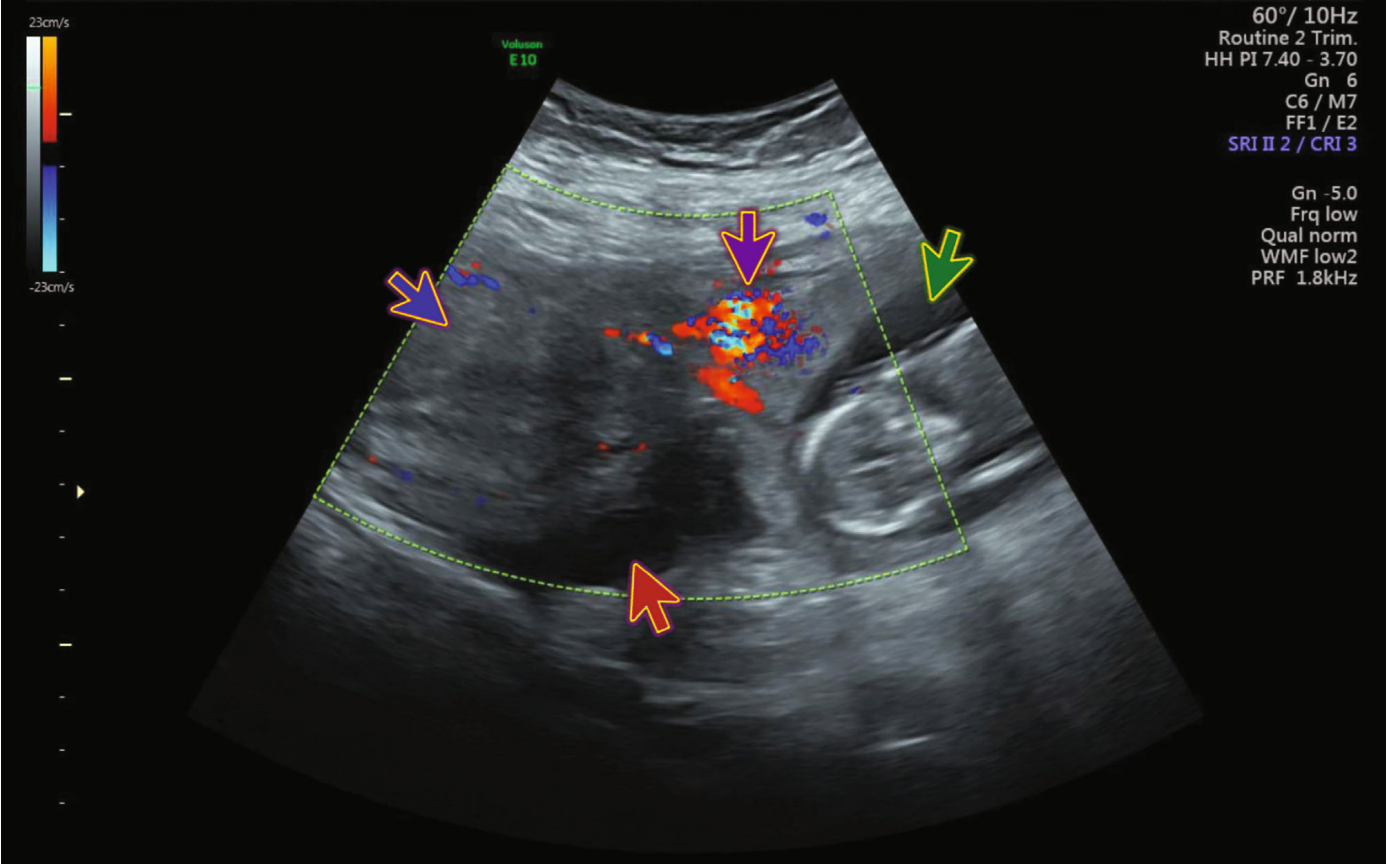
Ultrasound shows the gestational sac (green arrow) that was seen towards the left side of the uterus connected through a vascular stalk (purple arrow) to the uterus (blue arrow) and free fluid in the pelvis (red arrow).
